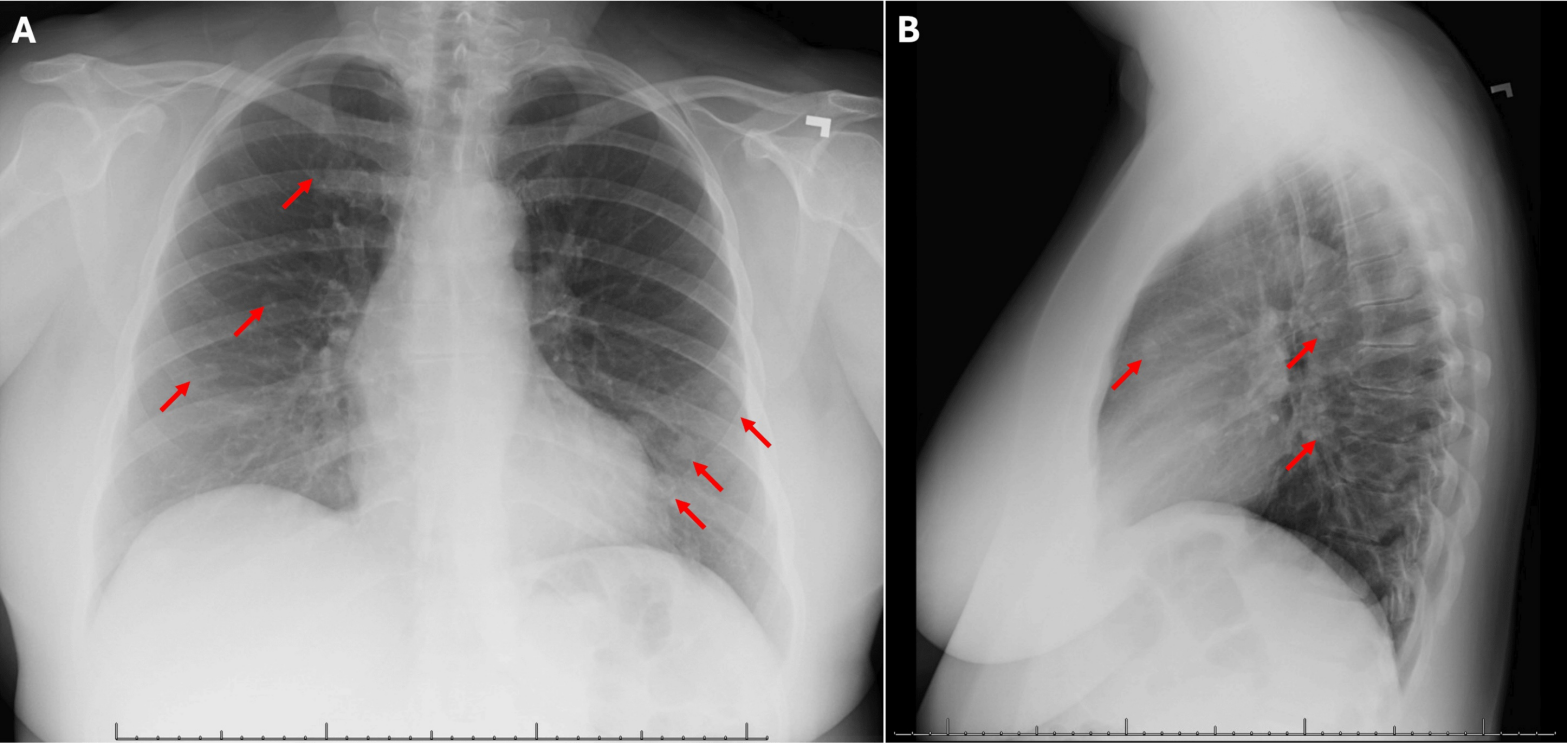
Chest X-ray imaging demonstrating diffuse bilateral pulmonary nodules. (a) Posterior anterior radiographic view. (b) Lateral radiographic view. Representative pulmonary nodules are indicated using red arrows.
